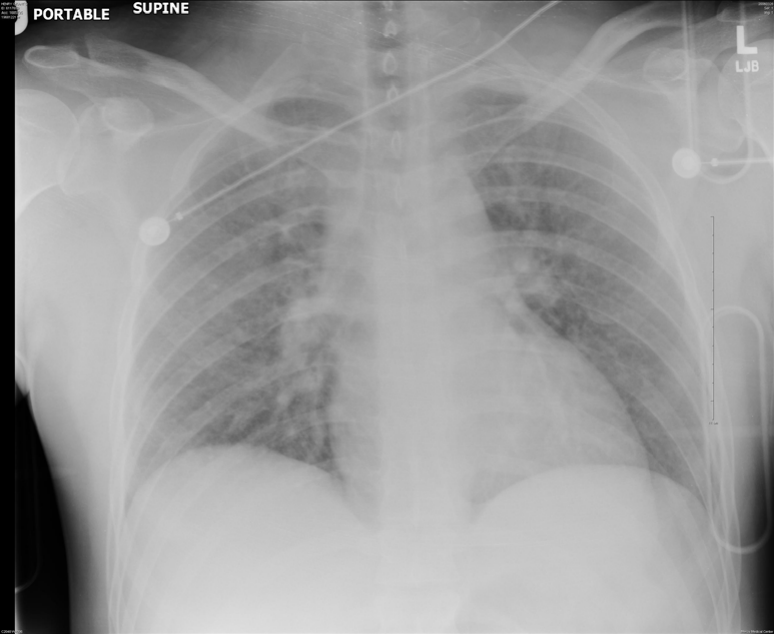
The chest radiograph taken in the trauma bay does not demonstrate acute intrathoracic injury.
